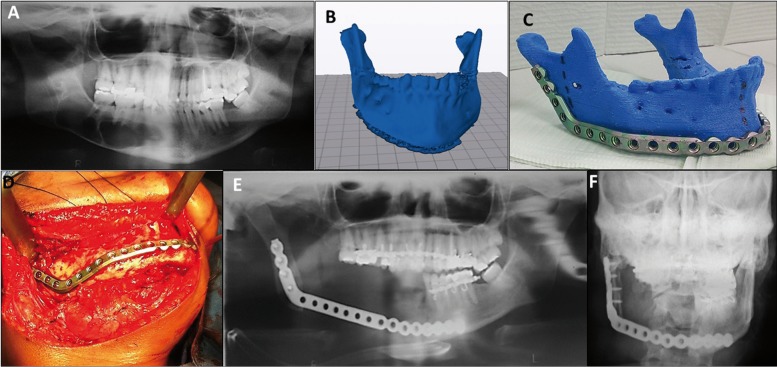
(A). Panorex x-ray showing a multilocular lesion, approximately 5.0 x 2.5 cm in size in the right mandible (B, C). MRP model created from patient DICOM data and reconstruction plate (2.7-mm mandible reconstruction plate (KLS Martin®, Jacksonville, FL, USA) prebended on its corresponding RMP. (D). Submandibular flap exposing the right mandible and tumor. (E, F) Postoperative panoramic and skull x-ray showing correct positioning of the reconstruction plate and mandibular symmetry.
